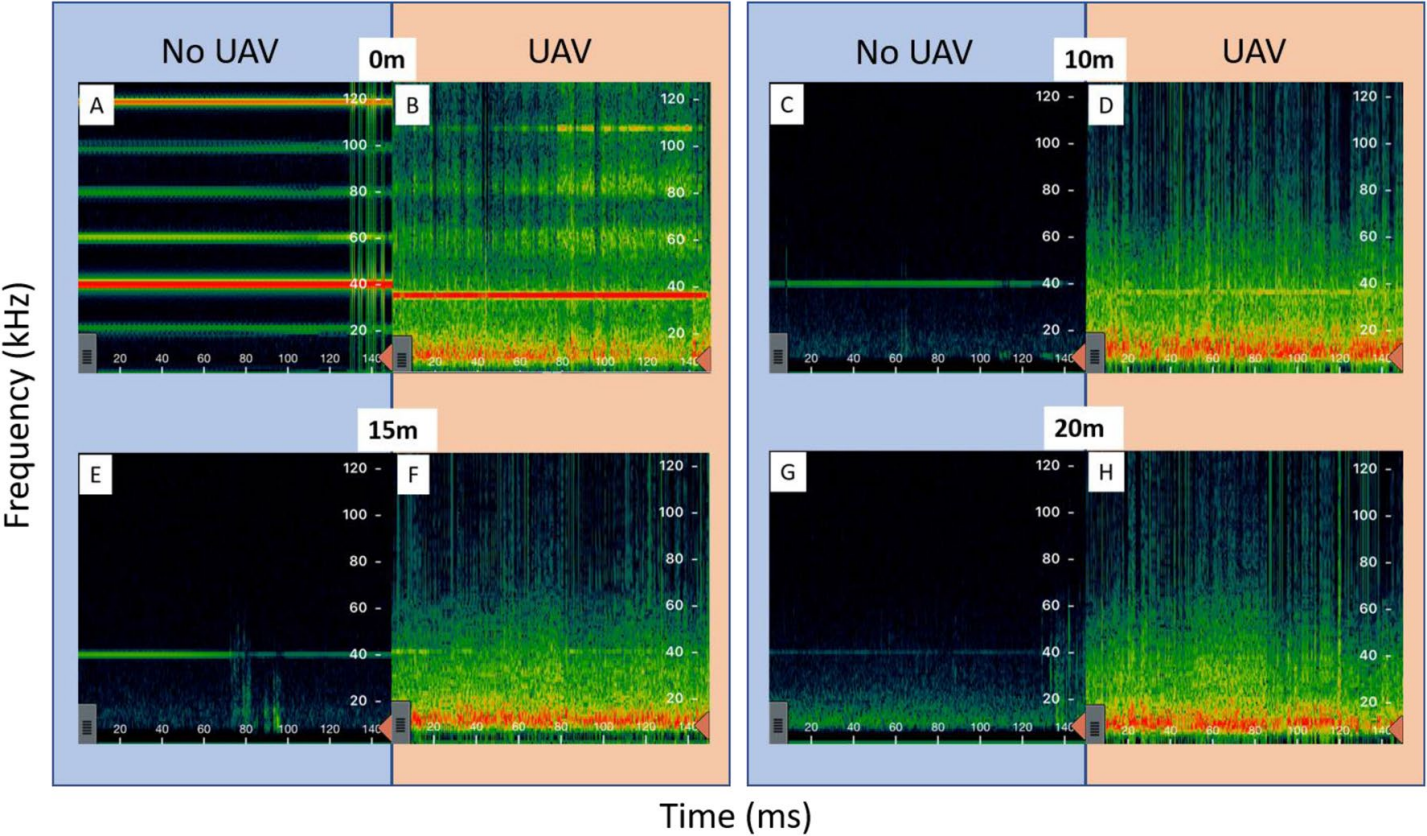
Visual comparison of signal detection range with ambient noise and UAV noise interference. Shading represents noise amplitude from low (green) to high (red). Panels inside the blue zones (A,C,E,G) represent samples taken under ambient noise interference, while panels inside the red zones (B,D,F,H) represent samples with UAV noise interference. Distance indicators represent the distance of the detector from the loudspeaker broadcasting ultrasounds. Bats present in our study area had calls typically between 25 and 80 Hz.
